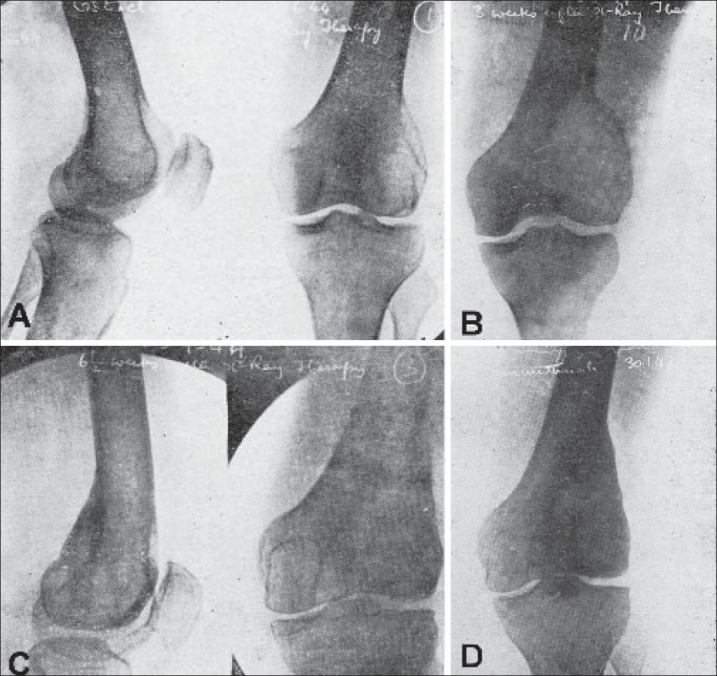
Effects of Irradiation in a case of osteoclastoma. (A) Before Roentgen irradiation. (B) 3 weeks after irradiation - Note increase in size and osteolysis. (C) 6½ weeks after irradiation - consolidation has commenced. (D) 2½ years after complete consolidation. (The patient failed to report between 6½ months and 2½ years and so pictures could not be obtained in this period
